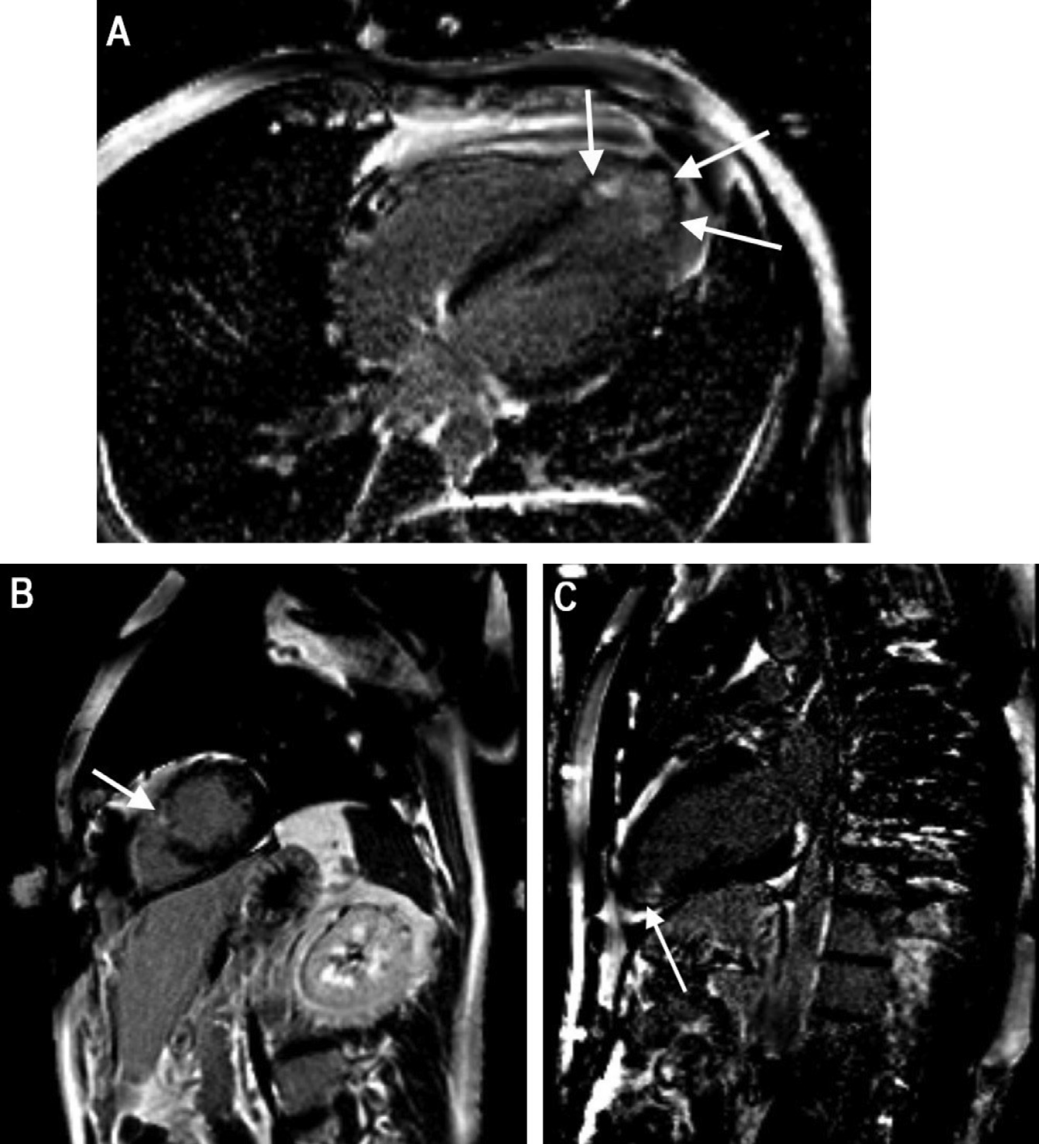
Cardiac magnetic resonance imaging shows (arrows) patchy mid-wall late gadolinium enhancement of the (A, B) left-ventricular apex, (C) septum, and free wall. The areas of late gadolinium enhancement are highlighted by the white arrows. These images (A-C) are phase-sensitive inversion recovery images.
